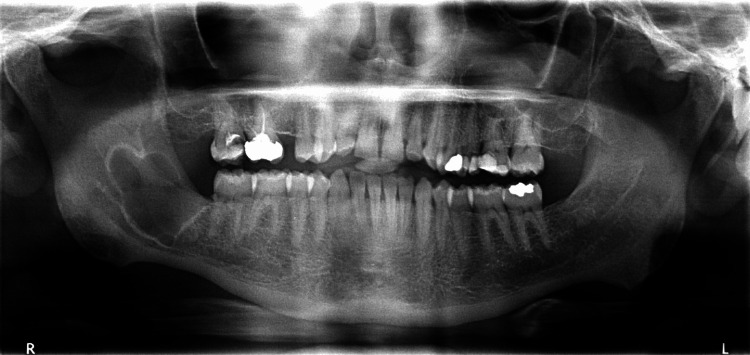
Radiographic imaging The orthopantomogram showed a well-circumscribed radiolucent lesion in the right posterior mandible.
